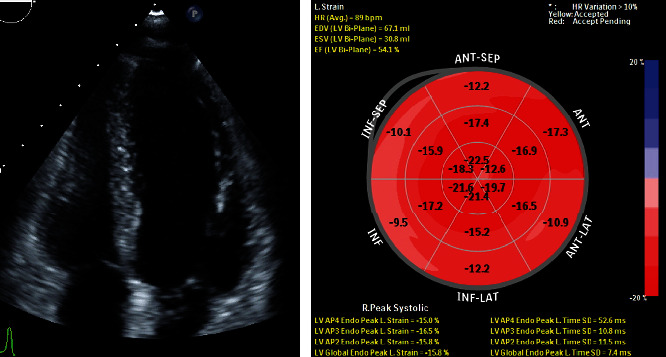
Echocardiographic examination at 6 months after patient's discharge from hospital.
